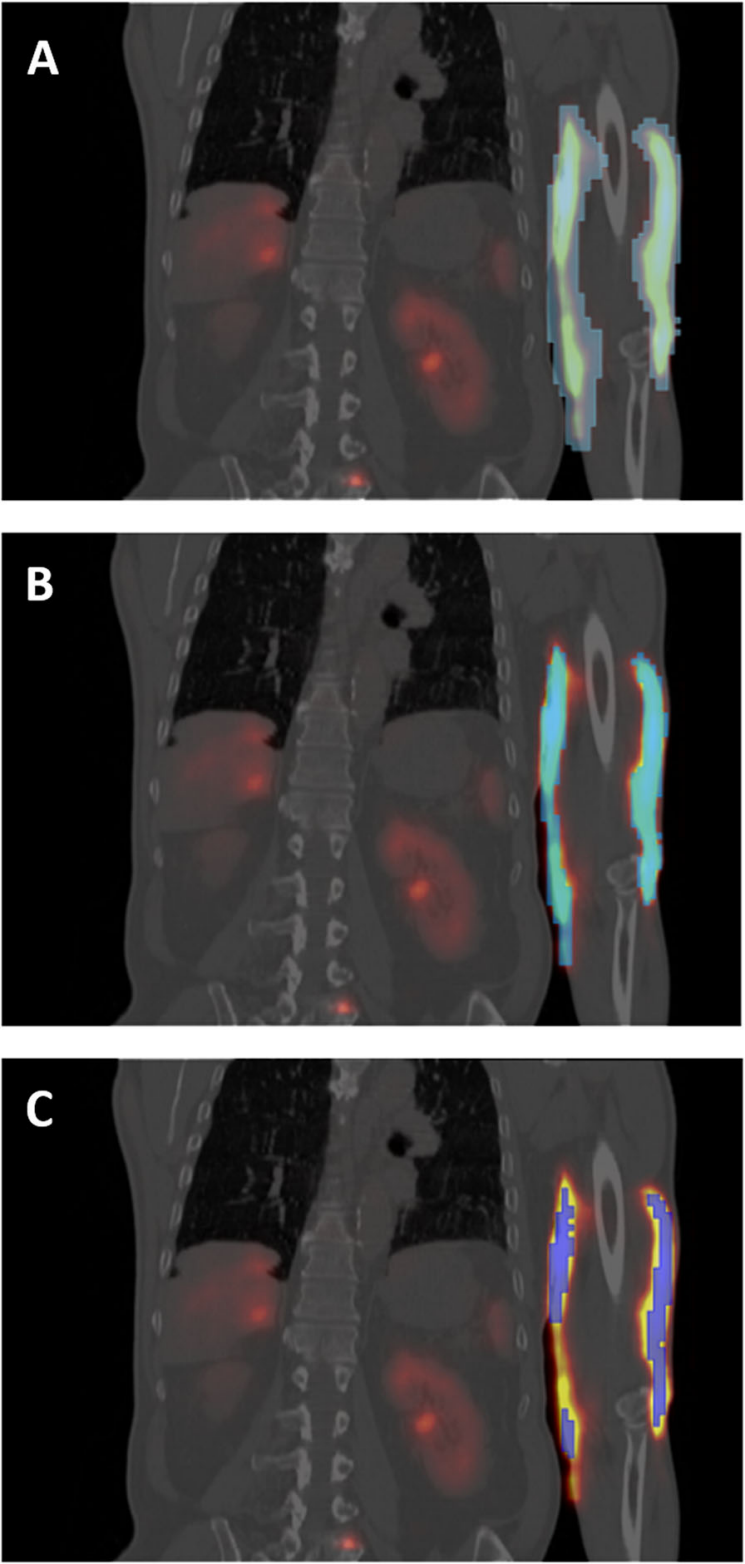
Coronal slice of *T*_5h_ SPECT/CT with superimposed VOI corresponding to 1000 mL (a), 400 mL (b), and 180 mL (c) VOIs
